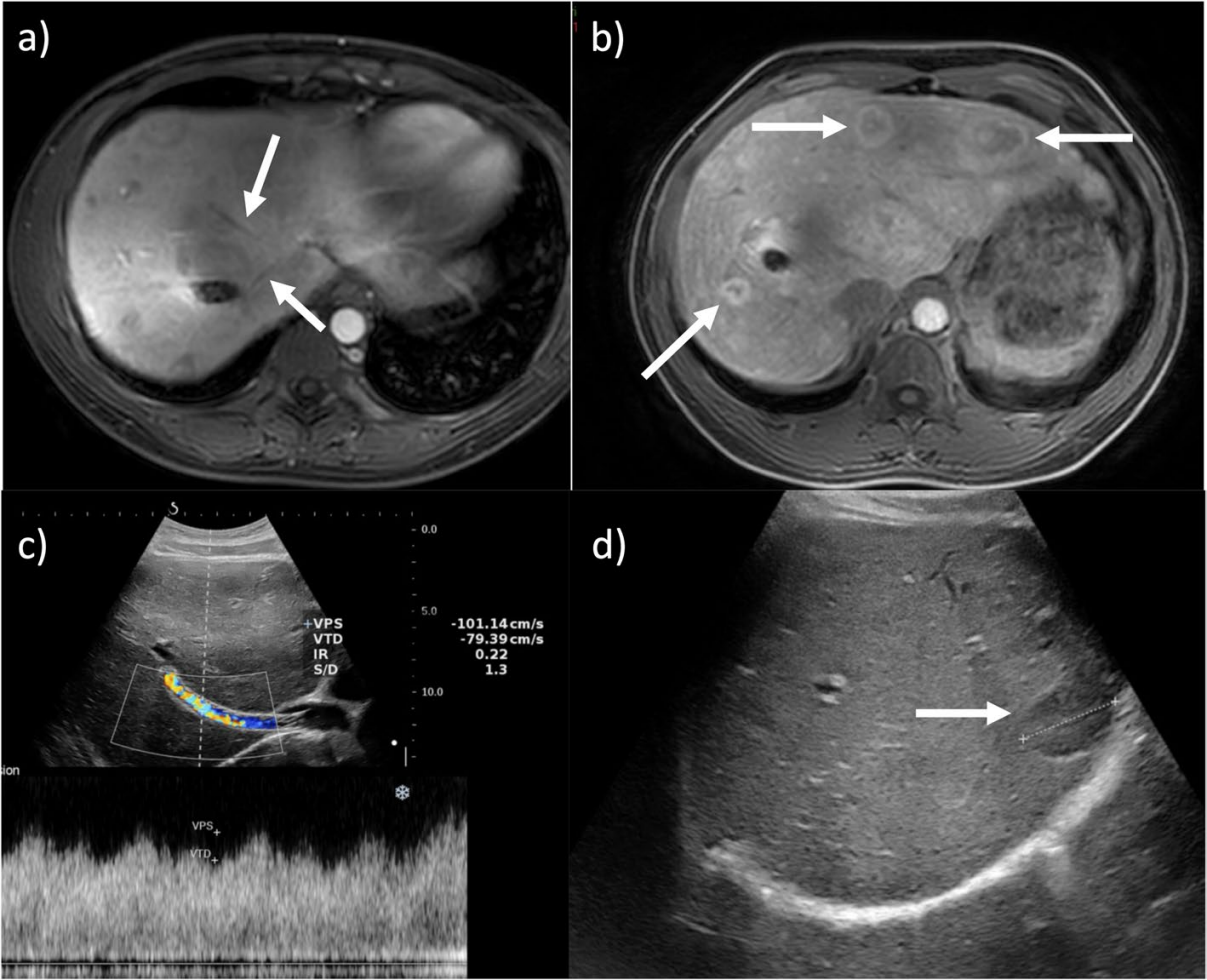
Ultrasound (US) surveillance during pregnancy in a 29-year-old woman with chronic Budd-Chiari syndrome. Pre-pregnancy gadobenate dimeglumine contrast-enhanced MR images (a, b) obtained during portal venous phase (a) and hepatobiliary phase (b) show the occlusion of the hepatic veins (a—arrows) and multiple benign regenerative nodules with peripheral hyperintensity on hepatobiliary phase (b—arrows). Note the presence of a trans-jugular intrahepatic portosystemic shunt (TIPS) connecting the superior vena cava and the portal system. US examination performed during pregnancy (c, d) allowed to evaluate the patency of the TIPS (c), to monitoring the lesion size (d) and to eliminate the presence of ascites
